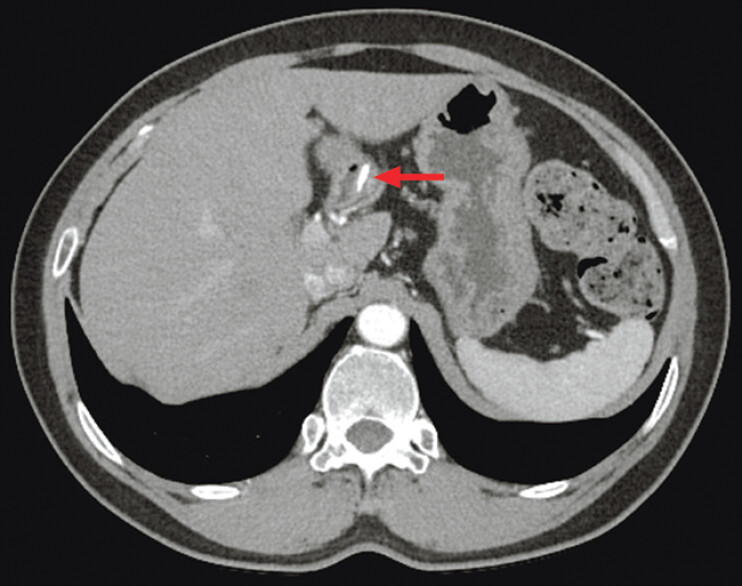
Abdominal CT showed a 13.9-mm-long spindle-shaped high density in the duodenal bulb.
